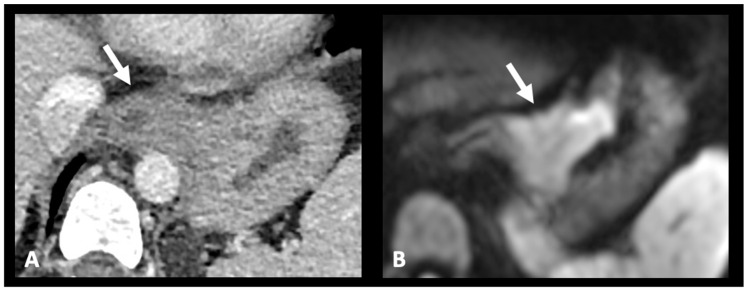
Contribution of MRI in assessing tumor location and extension. The transverse CE-CT image (A) shows a tumor at the gastroesophageal junction with its epicenter located above the cardia, classified as CT-Sievert 1. The MRI image on DWI (B) shows significant diffusion restriction at the level of the lesser gastric curvature, reclassifying this gastroesophageal junction tumor as a Sievert 3 tumor. DWI: diffusion-weighted imaging and CE-CT: contrast-enhanced computed tomography.
